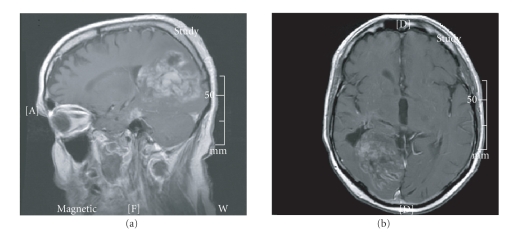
MRI brain T1 with gadolinium
